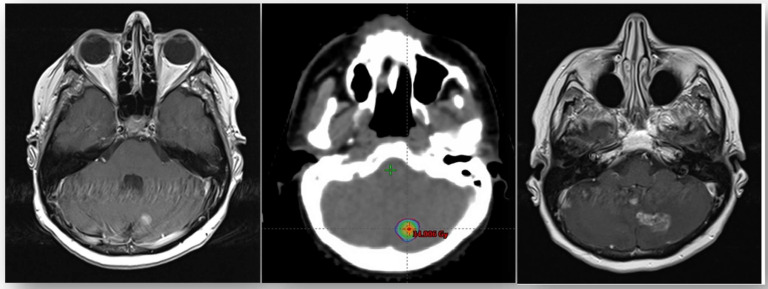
Brain MRI showing cerebral radionecrosis: (Left): T1-sequence MRI showing left cerebellar metastasis; (Middle) fSRT treatment planning; (Right) 12 months T1-sequence MRI: radionecrosis appearance. No other toxicity was experienced during treatment. No patient suspended PT.
